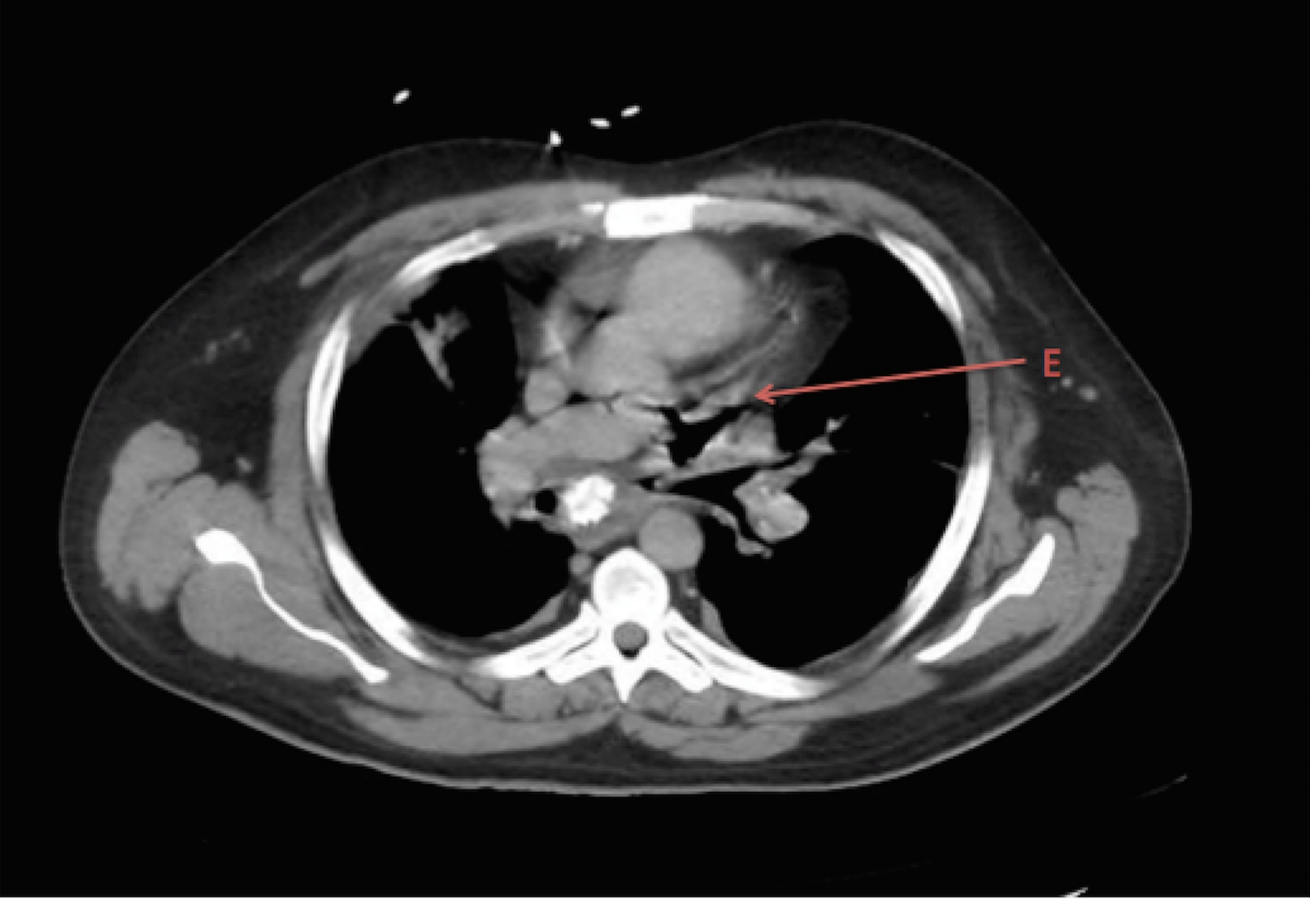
E: Axial plane CT scan showing fistulous tract between mediastinum, left upper pulmonary vein, and left mainstem bronchus
